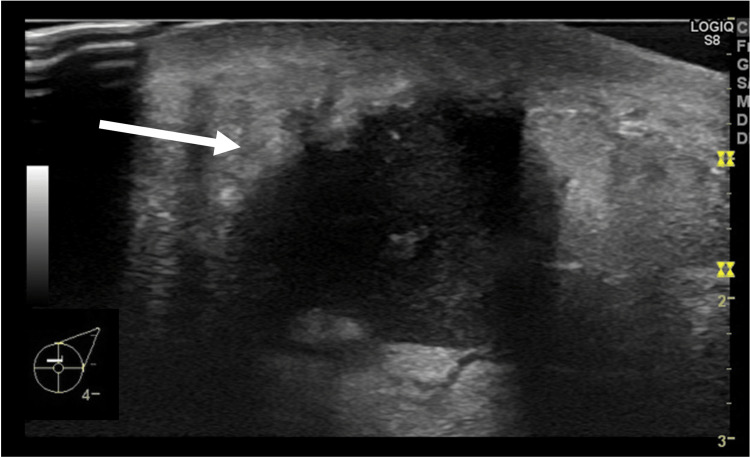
Breast ultrasound findings A 2.5 × 1.5 cm mass structure was observed. The lesions were mostly cystic, but some were solid. There was no halo, no rupture of the mammary border, and no evidence of blood flow (arrow).
